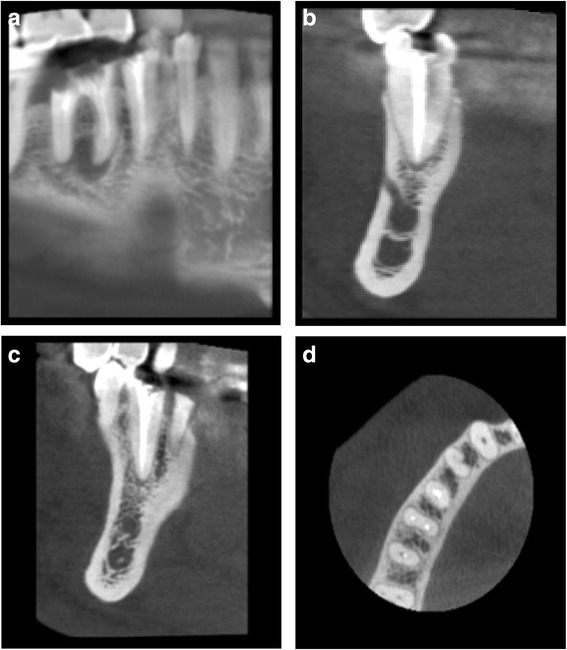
CBCT images of the extraction site. a Preoperative CBCT showing fractured and un-restorable teeth #45 and #46 planned to be extracted. b–d Cross sectional views
